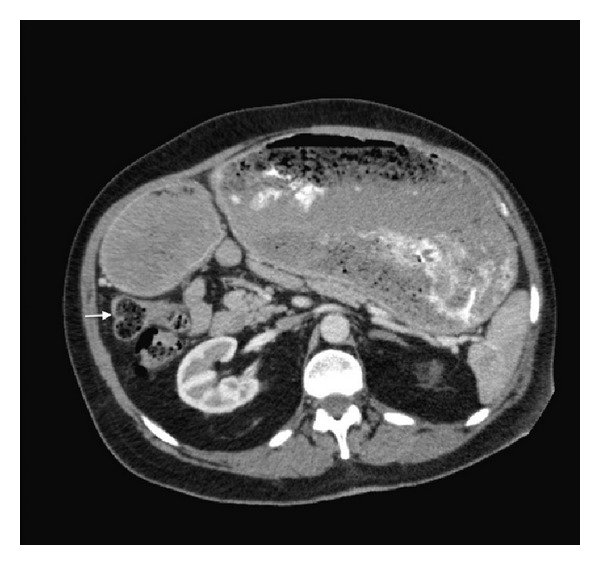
Coronal CT showing marked gastric distension with large amount of intraluminal gastric contents. A small bowel feces sign (arrow) is also appreciable in the region of the pylorus and the first portion of the proximal duodenum with surrounding adjacent inflammatory changes and mucosal wall thickening.
